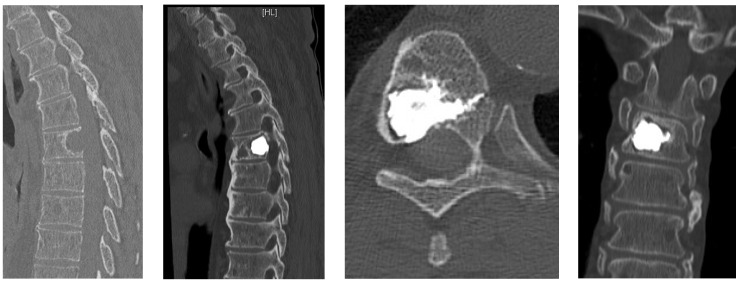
T8 kyphoplasty with intraoperative CT scan planning.
